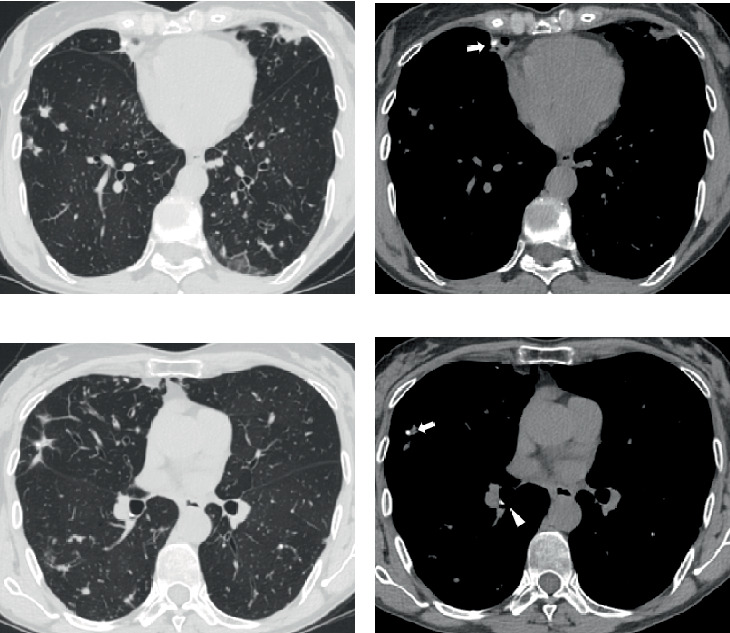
(a–d) 62-year-old woman, affected by bronchiectasis complicated by pulmonary NTM infection and broncholiths. High-resolution lung CT scan ((a, c) parenchymal window and (b, d) corresponding mediastinal window) shows multiple pulmonary nodules due to NTM infection and mild central bronchiectasis with mucus plugging (dilatation of the bronchial tree with mucoid impaction in a and c). Broncholiths are endobronchial (arrow in b and d) and peribronchial (arrowhead in d).
